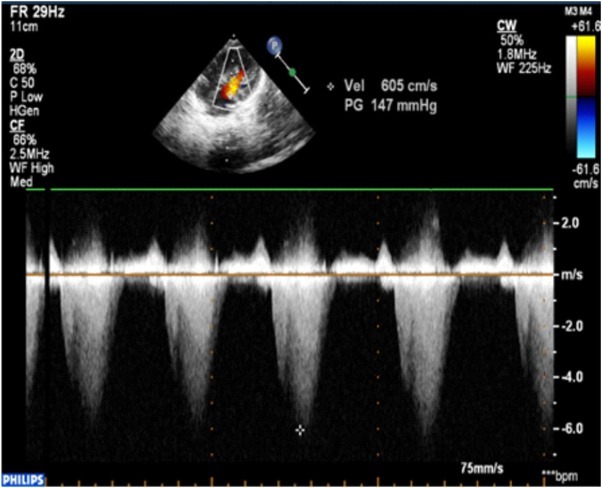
Continuous-wave Doppler apical 4 chambers view: hypertrophic cardiomyopathy predominantly septal with continuous-wave Doppler with a peak gradient in LVOT of 147 mmHg (LVOT-left ventricular outflow tract)
